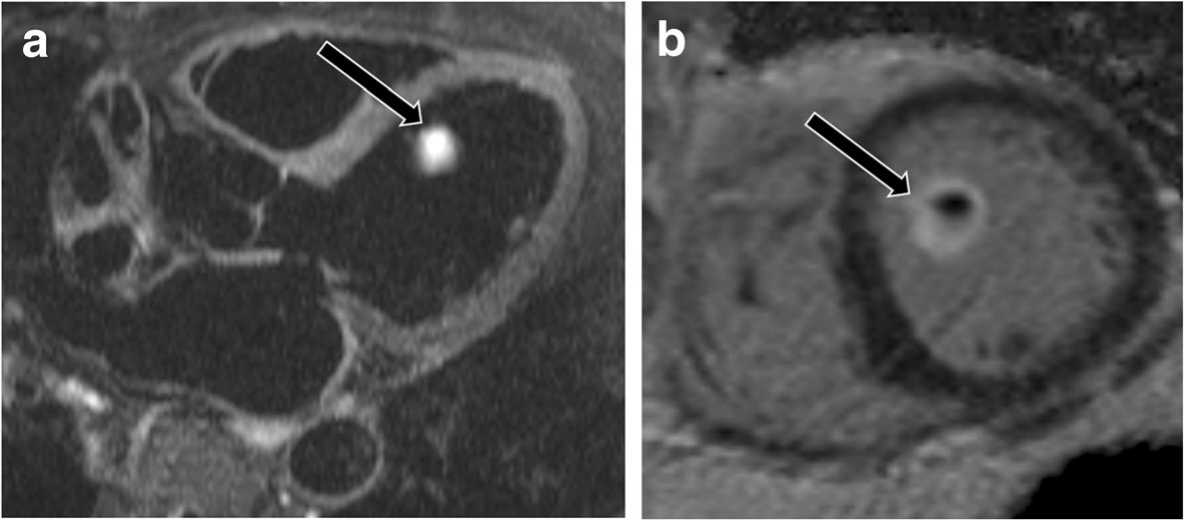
Fibroelastoma. a Three-chamber T2-weighted dark blood MR image shows a hyperintense mass attached to the anterolateral papillary muscle (arrow). b Short-axis delayed enhancement image shows delayed contrast enhancement (arrow). This was shown to be a fibroelastoma
